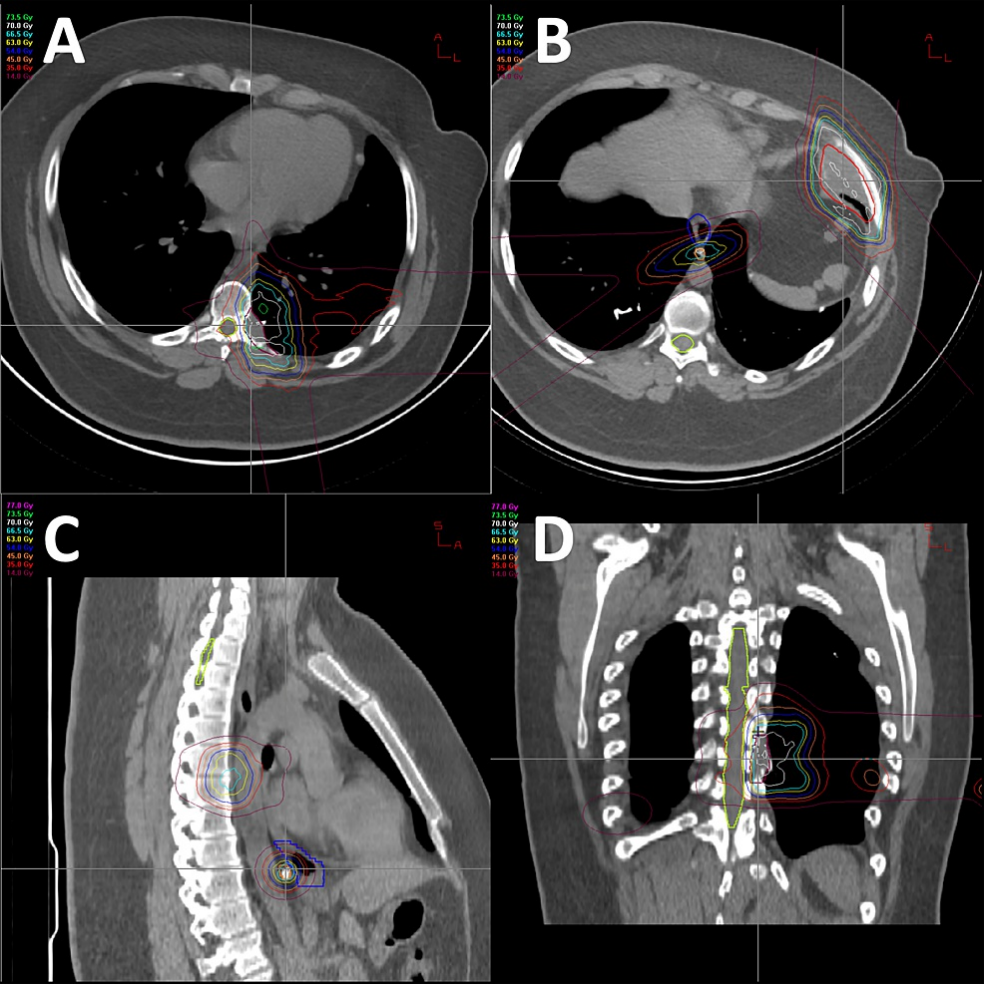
CT of the chest and radiation dose distributions A: CT of the chest axial view depicting radiation dose distributions targeting paraspinal lesion. B: CT of the chest axial view depicting radiation dose distributions targeting paraaortic and left anterior lower chest wall lesions. C: CT of the chest sagittal view depicting radiation dose distributions targeting paraaortic and paraspinal lesions. D: CT of the chest coronal view depicting radiation dose distributions targeting paraaortic, paraspinal, and mediastinal paraesophageal lesions
